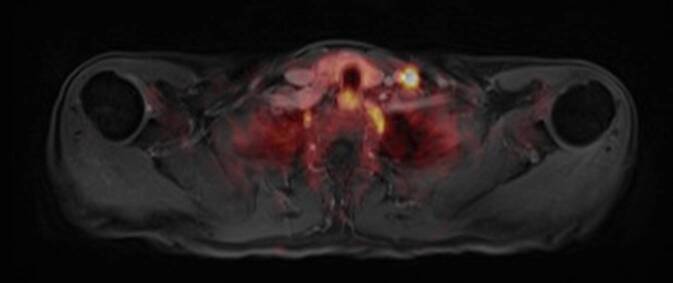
In der insgesamt blanden HNO-ärztlichen Routineuntersuchung waren zervikal keine pathologischen Lymphknoten palpabel. In der B‑Bild-Sonographie (Abb. 2) konnten in Region IV/V links 2 inhomogene lymphknotenähnliche Strukturen, rundlich konfiguriert mit einem maximalen Durchmesser von 1,2 cm, jeweils mit einem Solbiati-Index < 2, aber erhaltenem hilären Perfusionsmuster, dargestellt werden.
